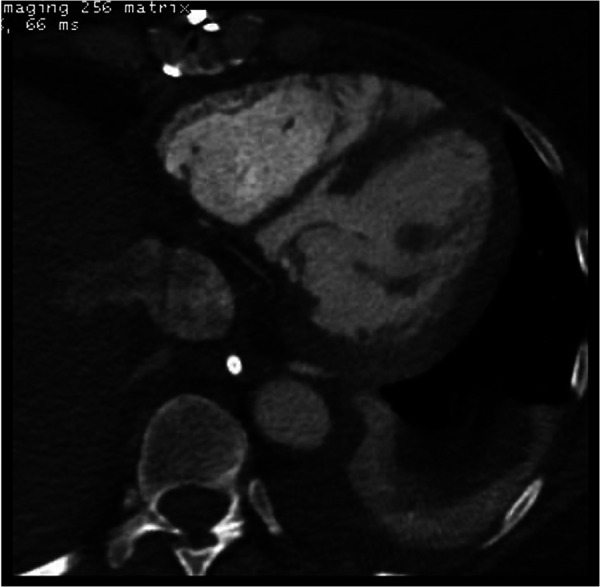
Contrast enhanced computed tomography of the chest performed 5 days after surgical repair of ventricular septal rupture (VSR) demonstrated the enlargement of small residual VSR.
